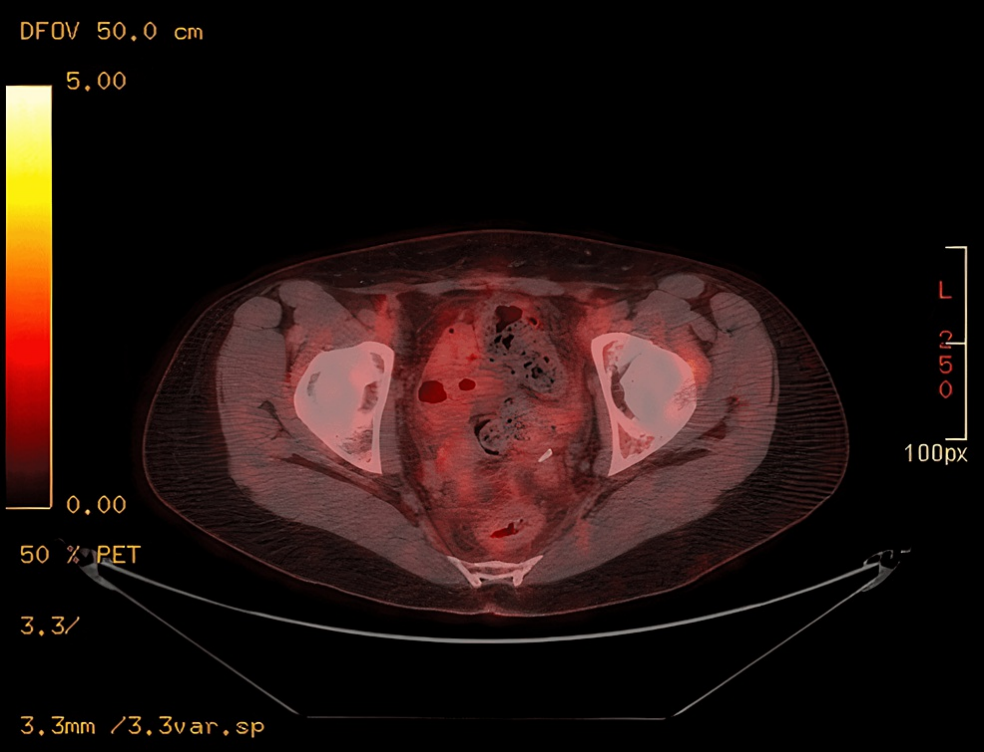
Follow-up PET-CT showing resolution of FDG-avid mass in the pelvis status post-hysterectomy. PET-CT: positron emission tomography-computed tomography; FDG: fluorodeoxyglucose
